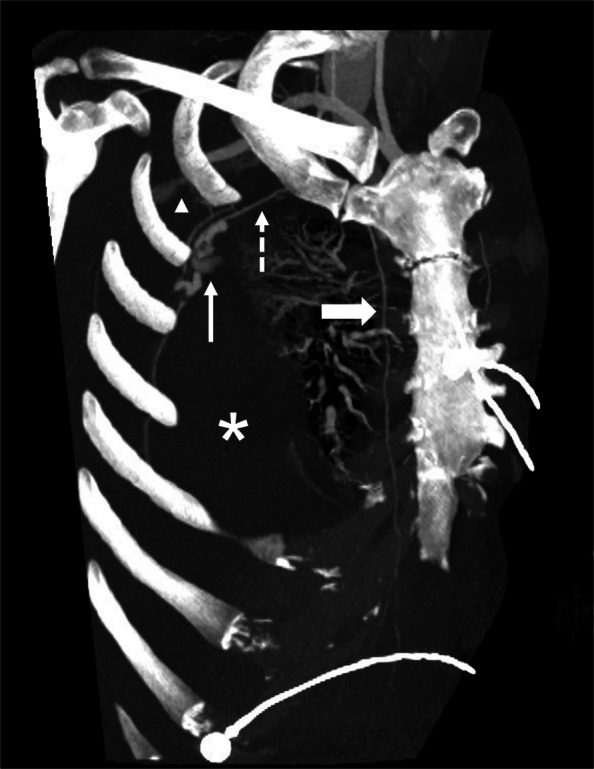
Multiplanar maximum intensity projection reformat image of the chest computed tomography demonstrating the internal thoracic artery (thick solid arrow), lateral costal artery (dashed arrow), intratumoral pseudoaneurysms (thin solid arrow), early filling axillary vein (arrowhead), and hemothorax (asterisk)
